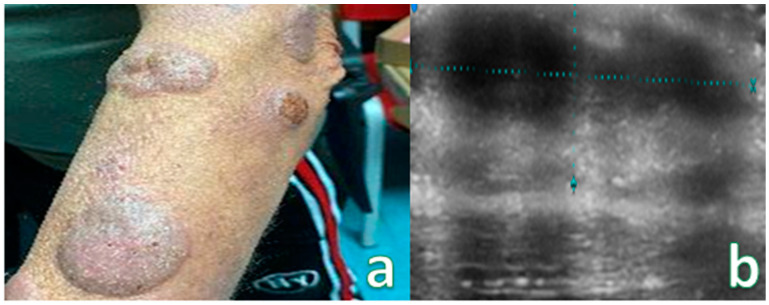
(a) Multiple pseudonodular lesion of the left forearm. (b) Examination performed with a very high-frequency ultrasound probe at 50 MHz in the anterior region of the left forearm showing iso-hypoechoic lesions with irregular margins located in the epidermal and subdermal layers highlighted by dashed blue lines. Images are low quality due to irregular surfaces of lesion.
